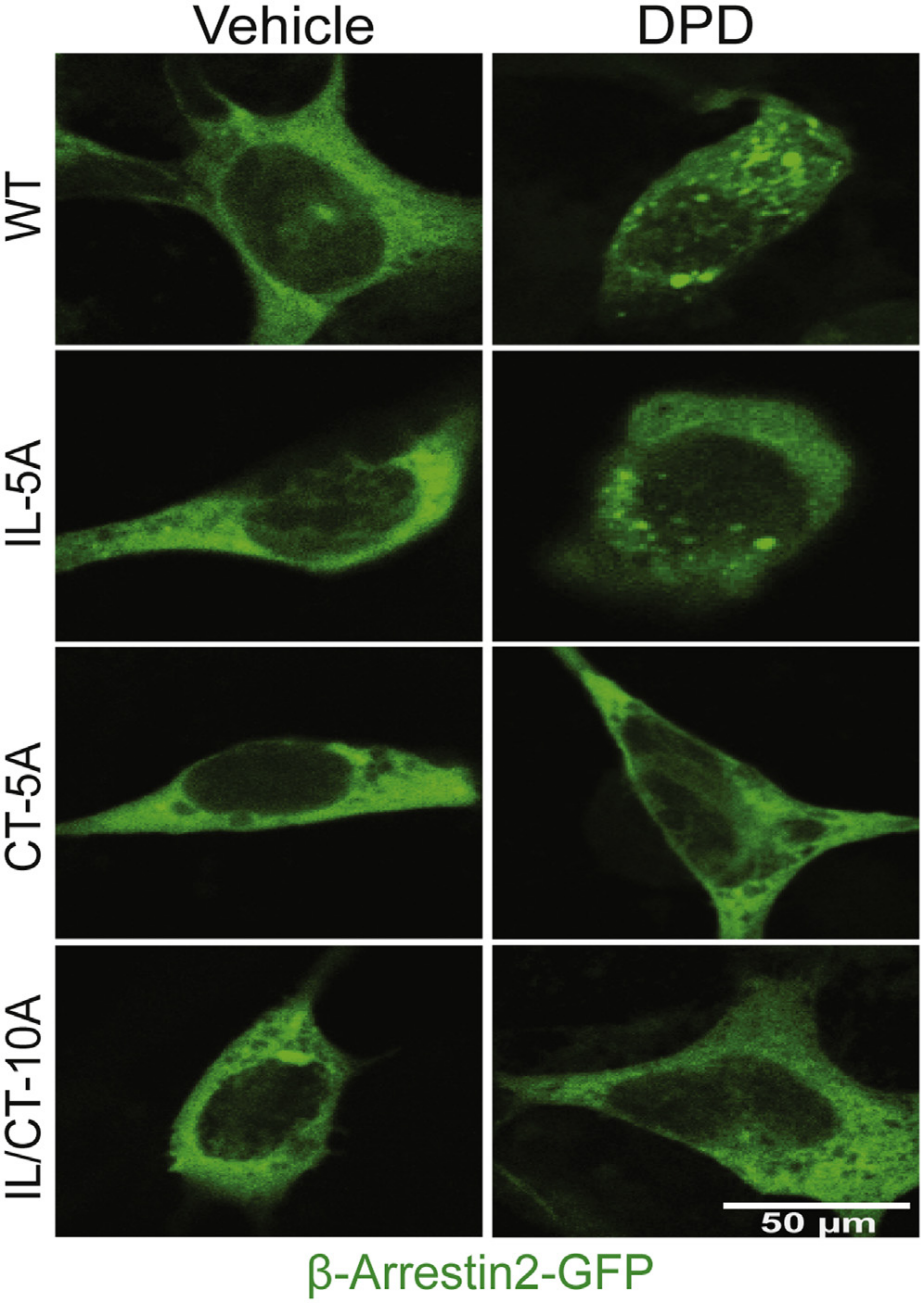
Qualitative assessment of agonist-promoted β-arrestin2 recruitment by WT and mutant TAS2R14 receptors. WT and IL-5A receptors exposed to agonist (10 min) displayed a redistribution of β-arrestin2–GFP from the cytosol to the cell membrane and a punctate accumulation pattern at the cell membrane. This appearance was not observed with CT-5A and IL/CT-10A receptors. Shown is a representative of five experiments performed. CT, carboxy-terminal tail; DPD, diphenhydramine; IL, intracellular loop; TAS2R14, bitter taste 2 receptor member 14.
